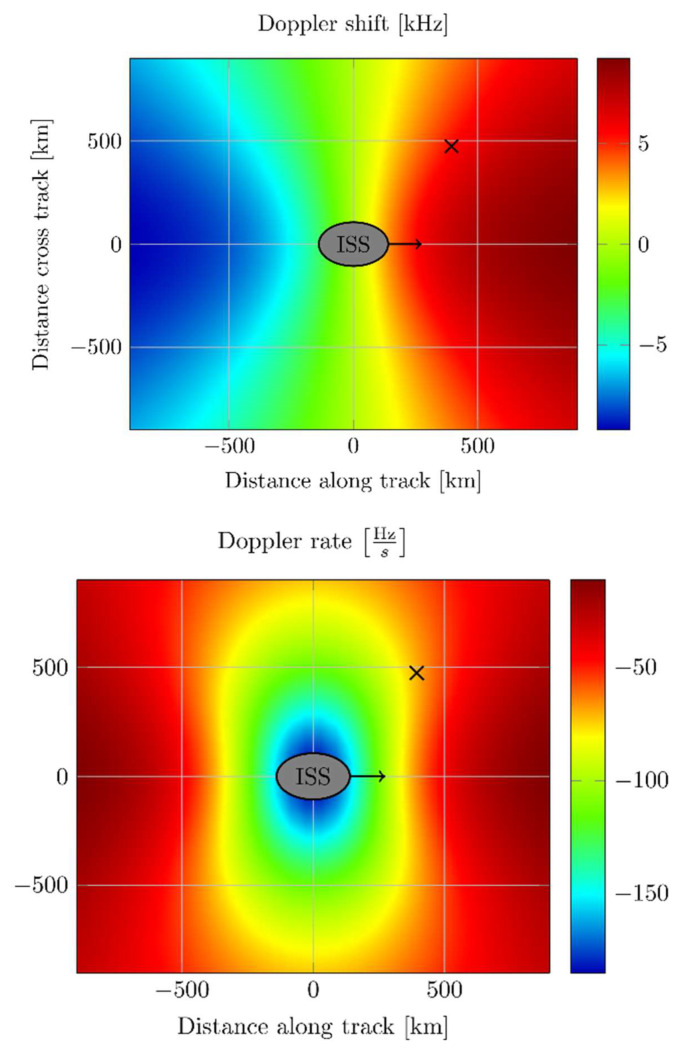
Doppler parameter of ICARUS.
